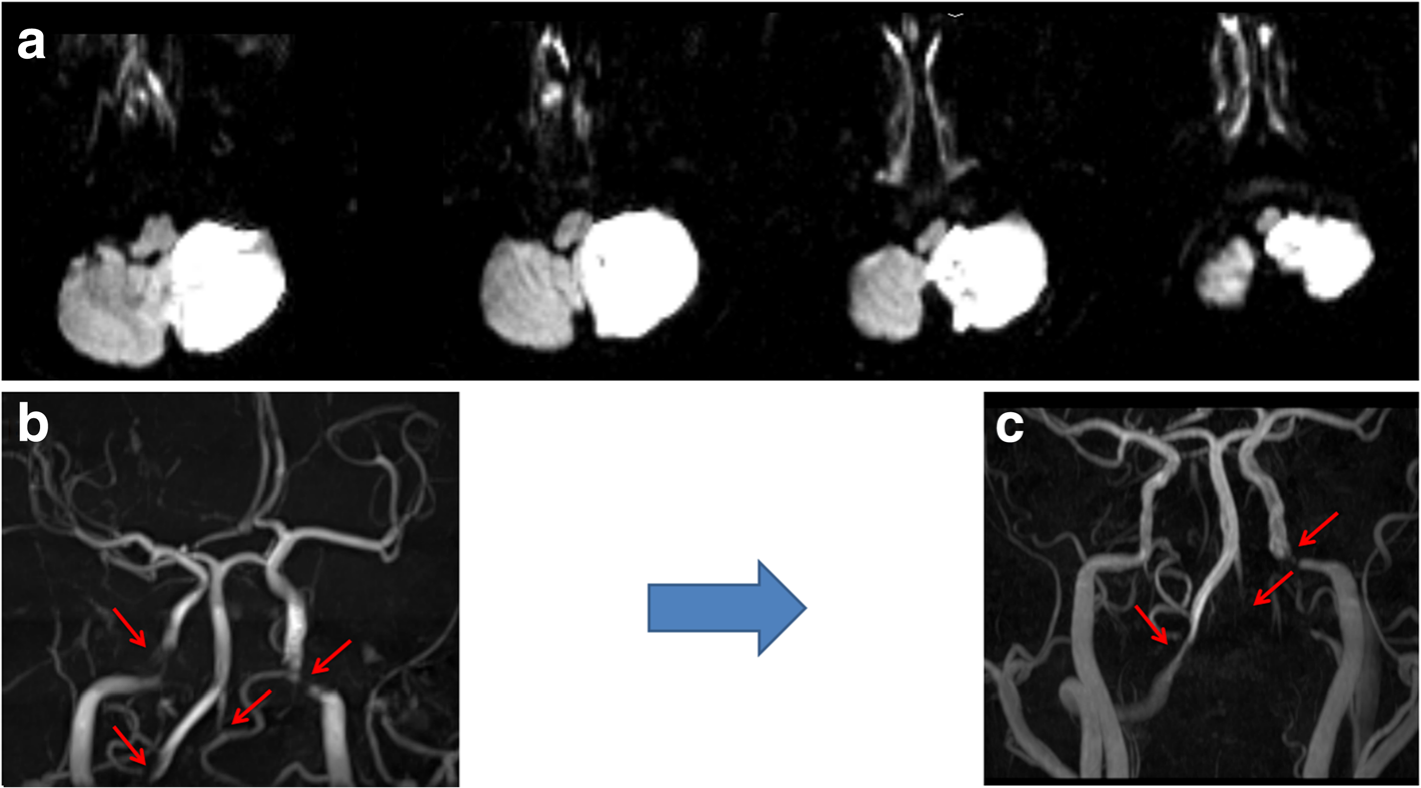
Diffusion weighted image (a) and time-of-flight magnetic resonance image initial (b) and at follow-up (c)
